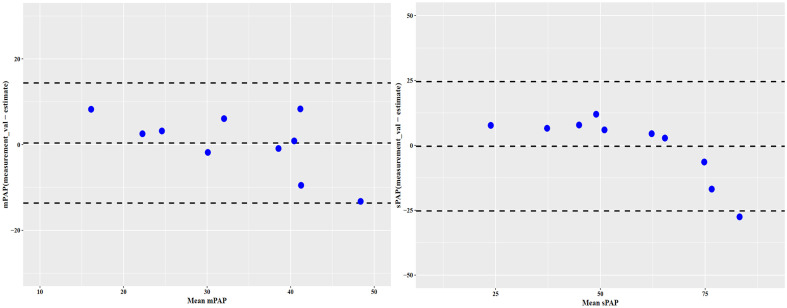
Bland-Altman plots comparing MRI pressure estimations (mPAP and sPAP) from the calculated model with pulmonary arterial pressures measured via RHC in the validation cohort.
